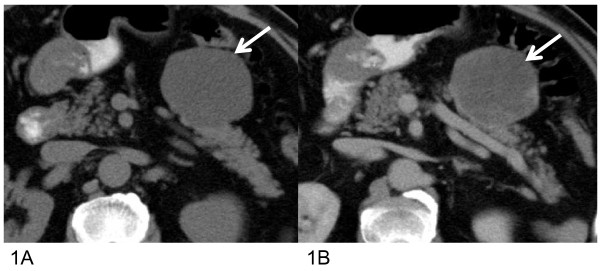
Computed tomography results. (a) Plain computed tomography (CT) shows a well-corcumscribed water-density (12HU) mass at the pancreatic body. (b) Contrast-enhanced CT shows the tumor with subtle enhancement (30HU).
